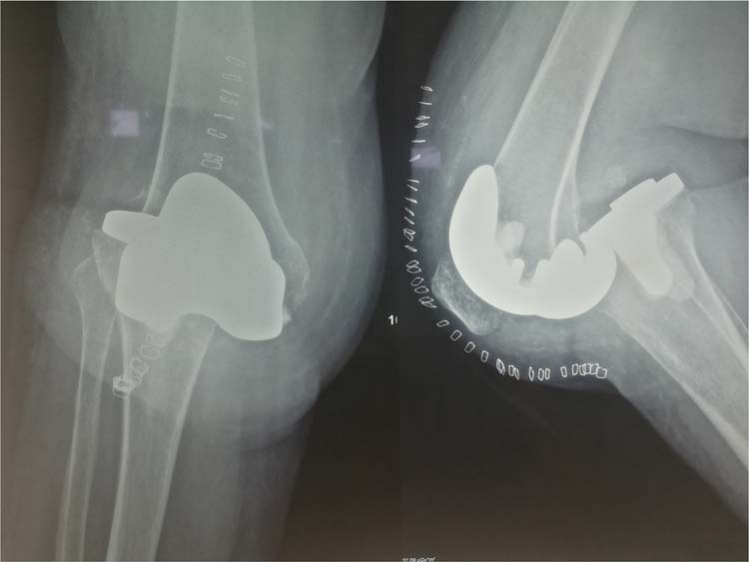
X-rays confirming the dislocation.
